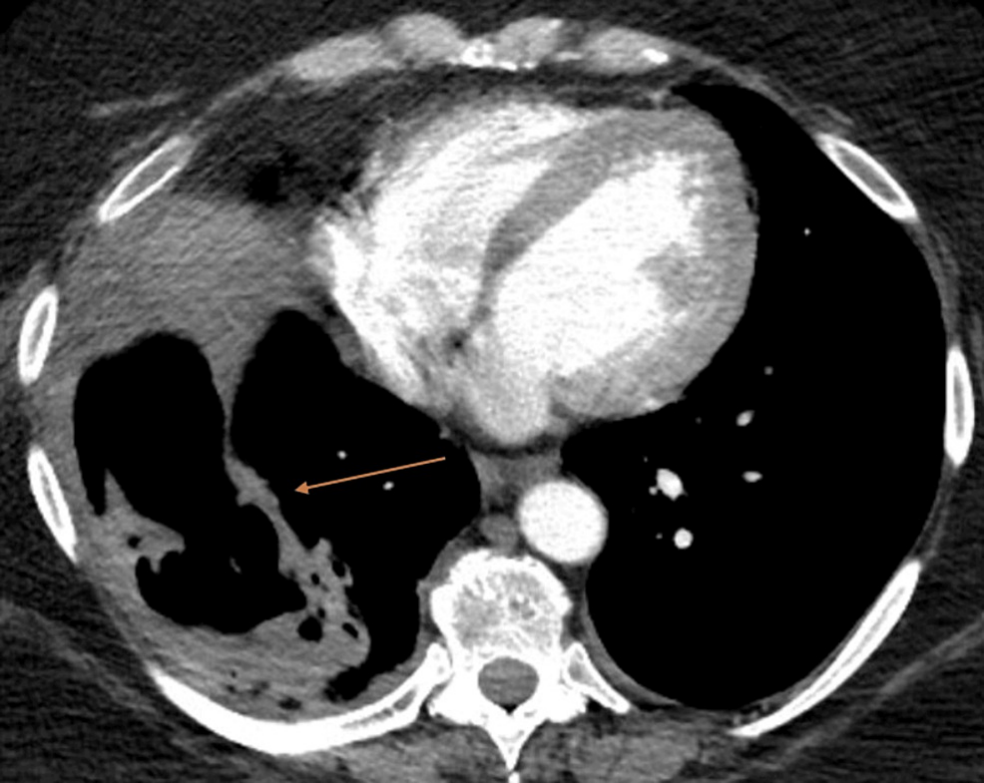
CT angiogram with a cavity in view Right lower lobe cavity seen. Fluid noted posteriorly. Anterior attenuation identified as diaphragm/superior portion of liver.
